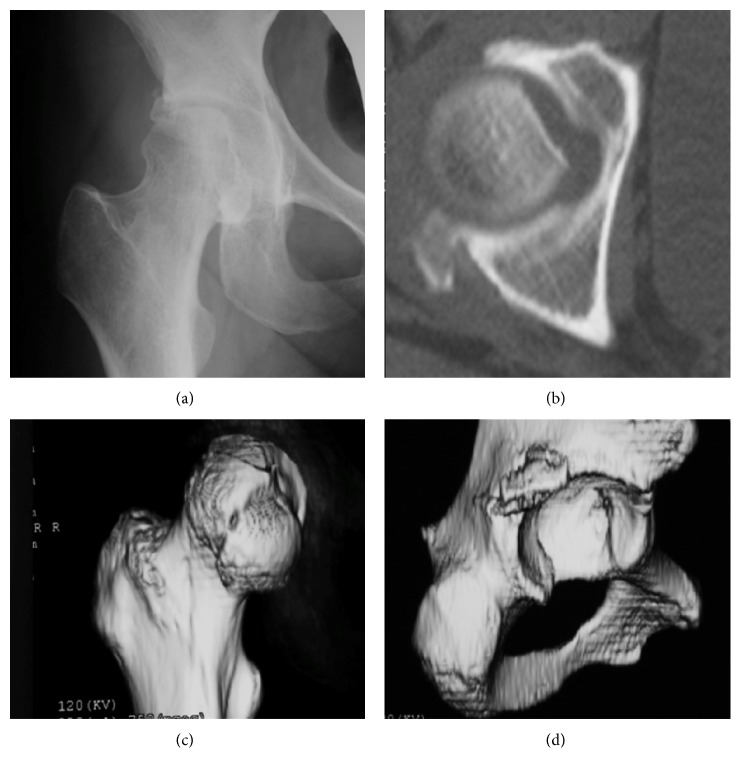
Case 2. A 45-year-old woman with Pipkin type-IV fracture of the right hip with posterior dislocation of the femoral head. (a) Anteroposterior X-ray image of the right hip after closed reduction, (b) axial computed tomography showing a defect of the femoral head and posterior wall of the acetabulum. (c) Three-dimensional image of the femur revealing displacement of a medial fragment of the femoral head. (d) Three-dimensional image of the acetabulum showing displacement of posterosuperior wall.
